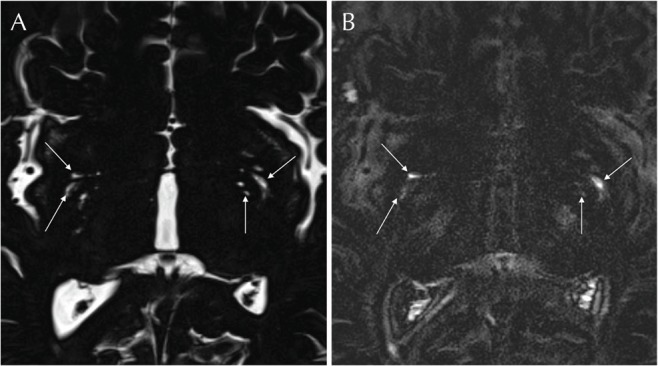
Reference images from another patient with endolymphatic hydrops (56-year-old man, estimated glomerular filtration rate (eGFR) of 64.6 mL/min/1.73 m^2^). Scan parameters were identical to those in Fig. 1. (A) Image from magnetic resonance (MR) cisternography shows multiple perivascular spaces in the bilateral basal ganglia. (B) Heavily T_2_-weighted three dimensional-Fluid attenuated inversion recovery (3D-FLAIR) obtained four hrs after intravenous single dose administration of gadolinium based contrast agent (IV-SD-GBCA). High signal intensity is observed in the bilateral perivascular spaces in the basal ganglia (arrows).
